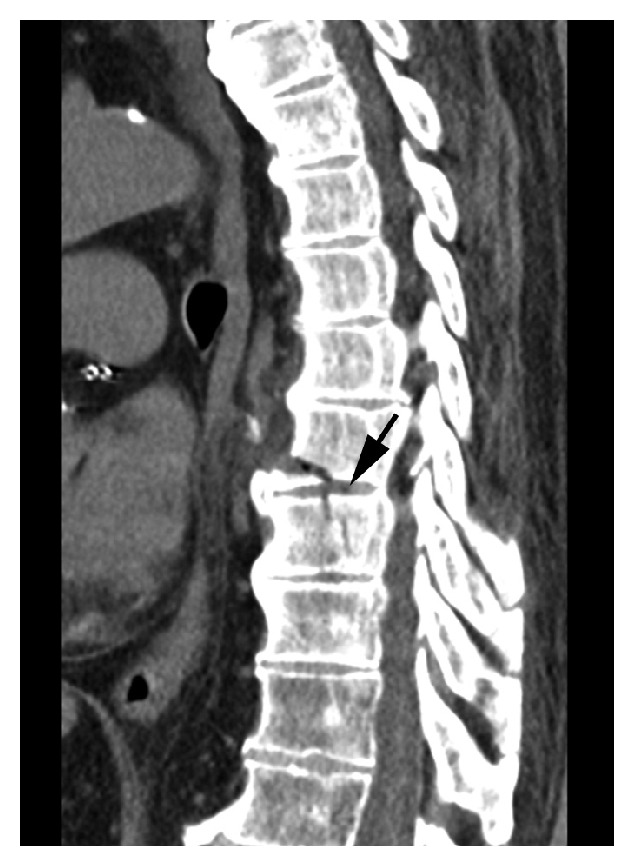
Sagittal CT scan of the thoracic spine showing an oblique fracture traversing the T8 inferior endplate, T8-T9 interspace, and posterior aspect of the T9 vertebral body (*arrow*).
